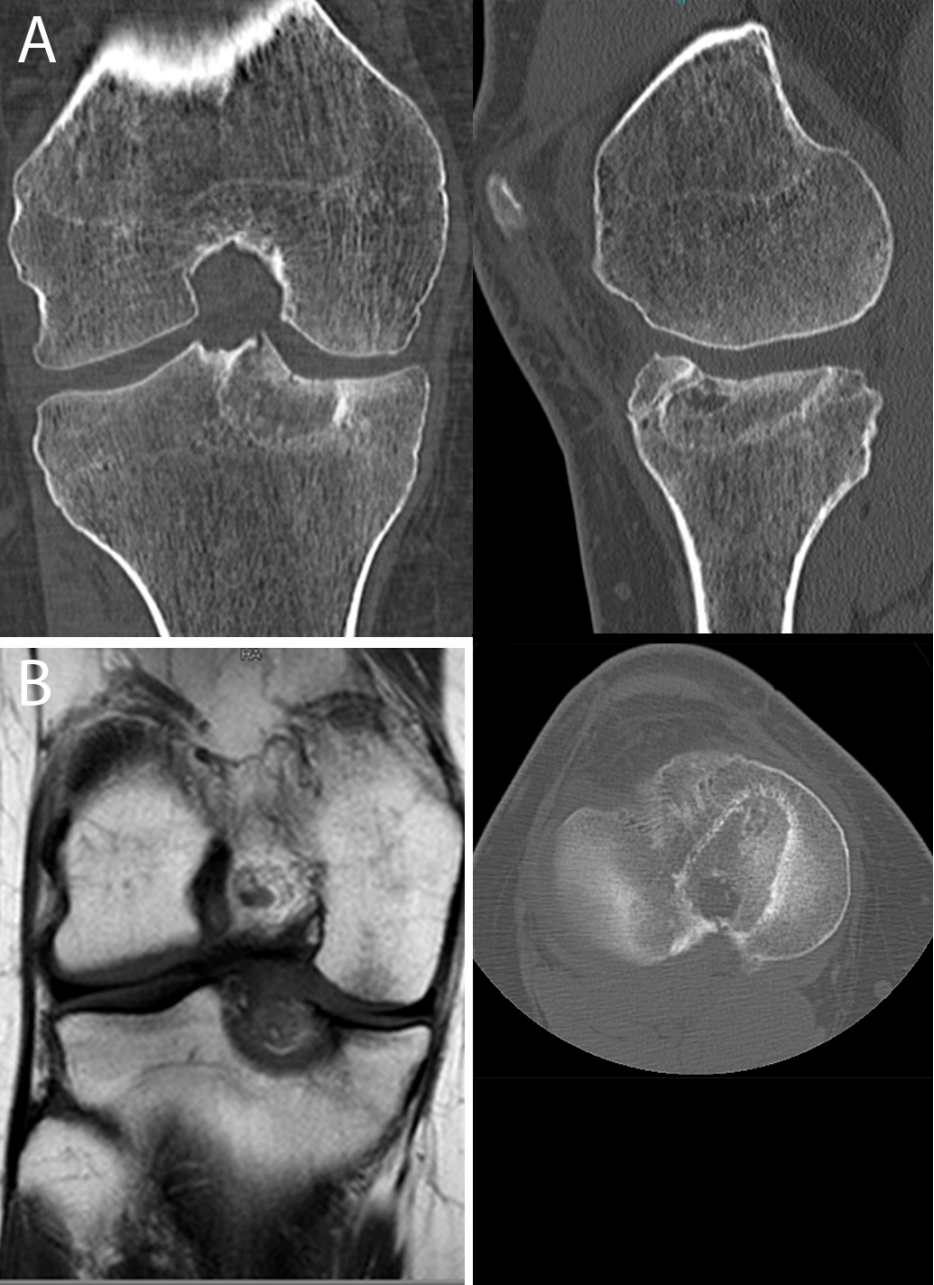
A, B: CT and MRI scan performed at one year of follow-up.
